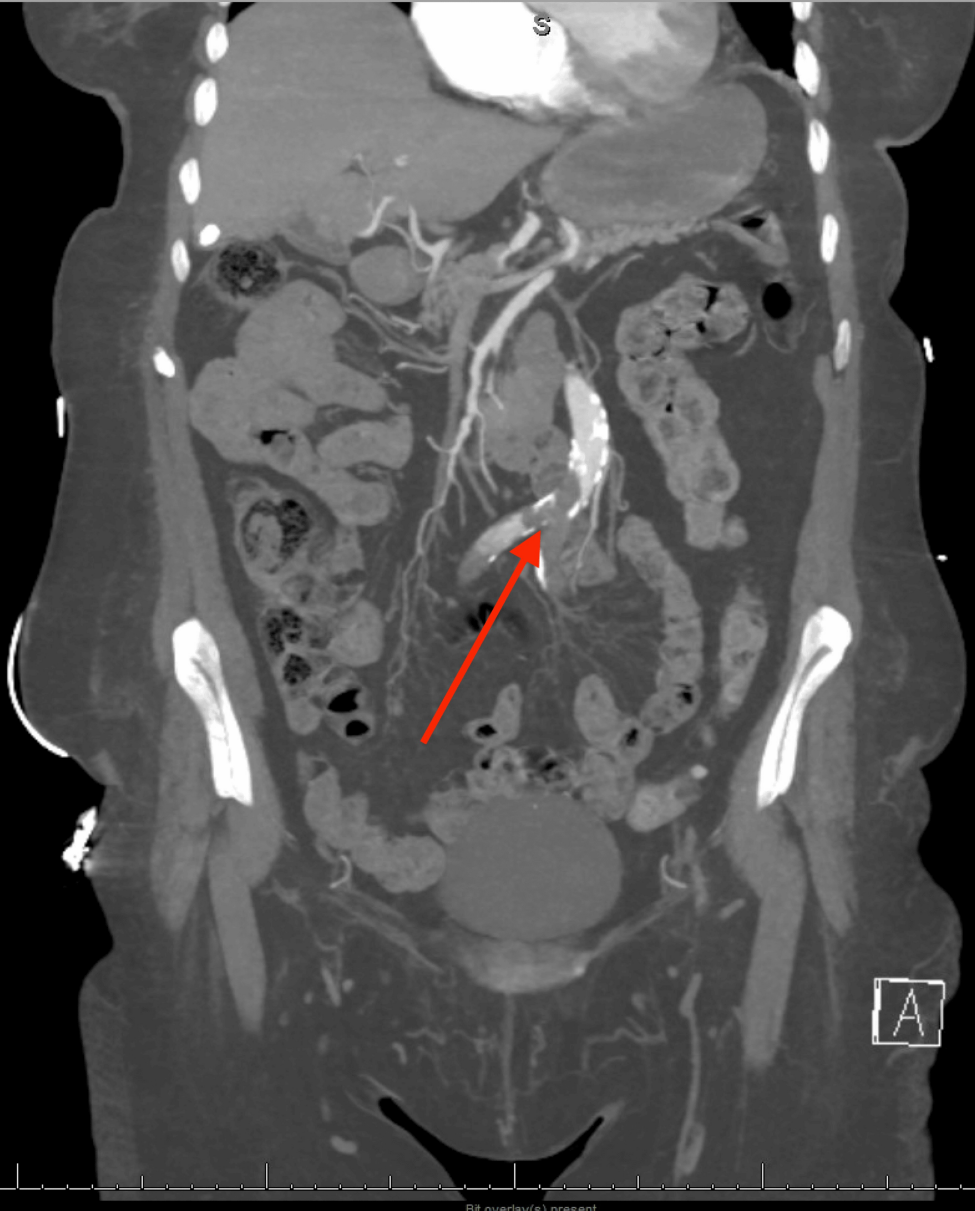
Similarly, computed tomography angiography of the abdomen on a coronal slice redemonstrated the 3 cm distal aortic occlusion, demonstrating the distal aorta occluded just above the bifurcation over a 3 cm segment by thrombus/plaque and extending into bilateral proximal common iliac arteries with distal reconstitution. The red arrow denotes a 3-cm distal aortic occlusion from plaque/thrombus.
